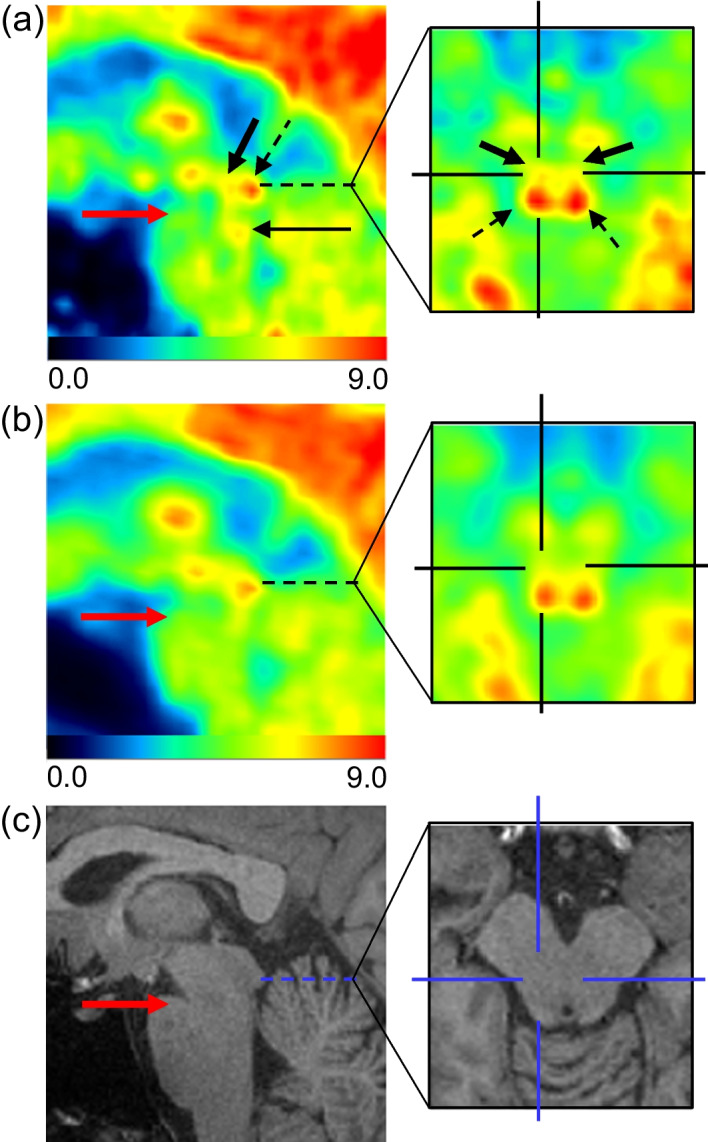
Sagittal views of the brainstem using Vrain (a), whole-body PET (b), and MRI (c). The right expanded images show the axial images at the level (dashed line in the sagittal image), which is 4 mm above the isthmus (red arrow). The midbrain part of the raphe nucleus (bold arrows) is depicted ventral to the inferior colliculus (dashed arrows) using Vrain (a), and the centre of the crossbar is placed at the right component of the raphe nucleus in the midbrain. The pontine part of the raphe nucleus is also visualised on sagittal view (black arrow) (a) at 2 mm below the isthmus (red arrow). The raphe nucleus is not visualised on the whole-body PET (b). The SUV is indicated by the colour scale
